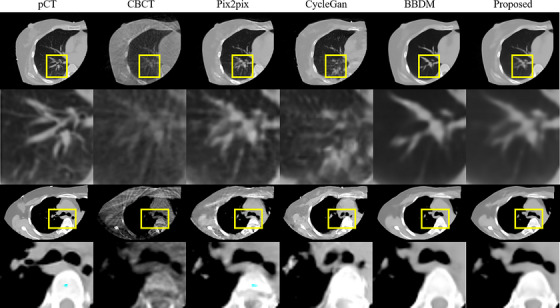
Qualitative comparison against representative baseline methods. The Proposed method avoids the high‐frequency artifacts of Pix2pix, the significant blurring of CycleGAN, and the over‐smoothing of fine details observed in BBDM. It demonstrates a superior ability to render the daily CBCT's anatomy with the high‐contrast quality of a pCT, successfully suppressing noise while preserving critical structural details, including small lung nodules and cardiac vessels. All images are displayed with a window setting of [‐1000, 700] HU.
